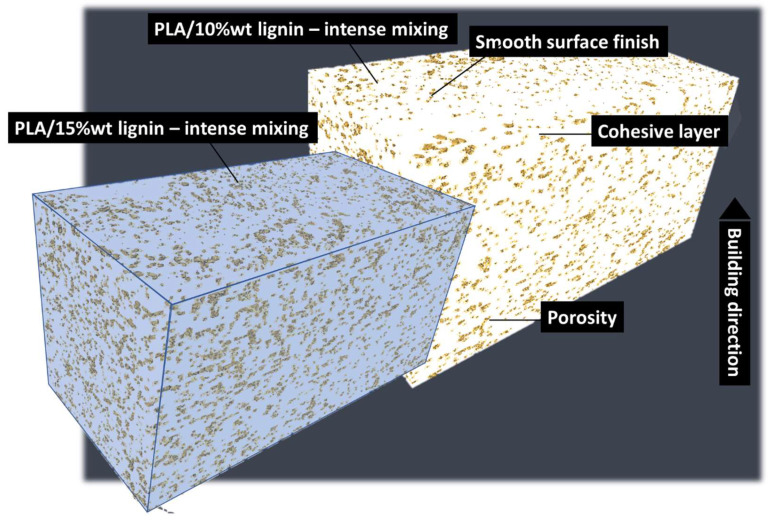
X-ray microtomography results: comparison between the microstructural features of 3D-printed cured acrylate-modified PLA/10 wt% and 15 wt% lignin.
